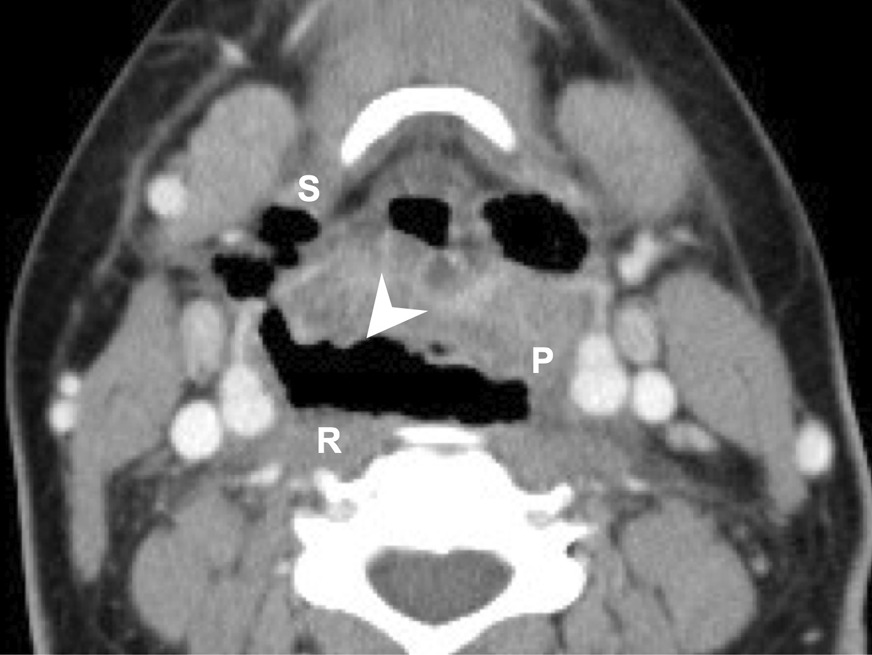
The axial CT view of a patient with a deep neck infection and esophageal perforation. *R* retropharyngeal space; *P* parapharyngeal space; *S* submandibular space; air dissection (arrowhead)
